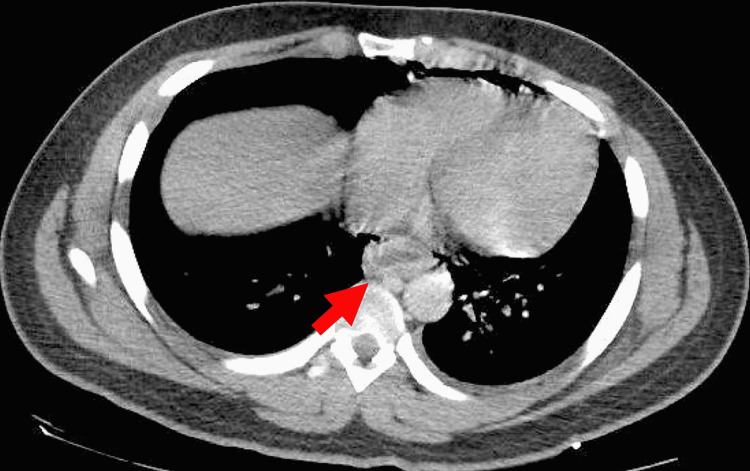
Transverse-view computed tomography of the chest in lung window Red arrow demonstrates esophageal thickening and perforation secondary to Boerhaave syndrome.
